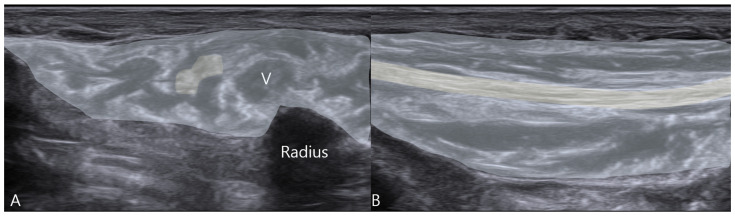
Post-hydrodissection ultrasound imaging of the superficial radial nerve: (A) short-axis view showing the nerve (yellow region) and the distribution of injected D5W fluid (blue shaded region), indicating nerve decompression; (B) long-axis view confirming expanded perineural space with hydrodissection fluid around the nerve.
